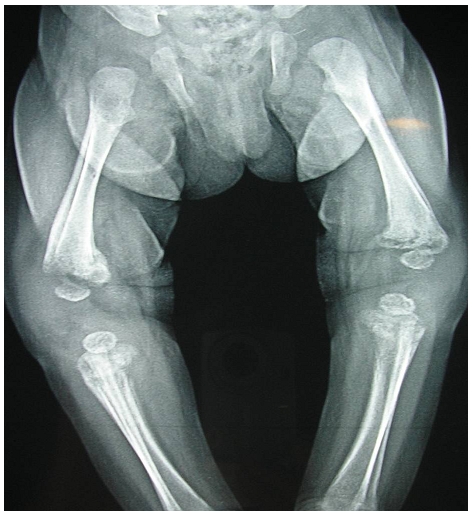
Radiographie montrant une ostéolyse des genoux et une périostite chez un 2 mois et 22 jours pris en charge pour syphilis congénitale révélée par une fracture spontanée
